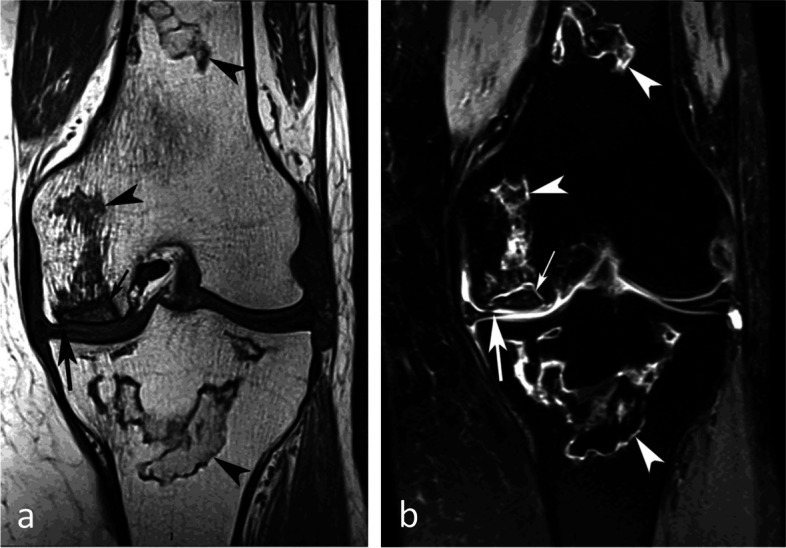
MRI pattern of osteonecrosis of systemic origin. a, b T1-weighted (a) and fatsuppressed T2-weighted MR images (b) show disseminated areas of osteonecrosis surrounded by linear rims appearing in low or high signal intensity on T1- or T2-weighted images, respectively (arrowheads). A necrotic collapsed area is shown, with fracture in the corresponding intraosseous lesion (arrows)
